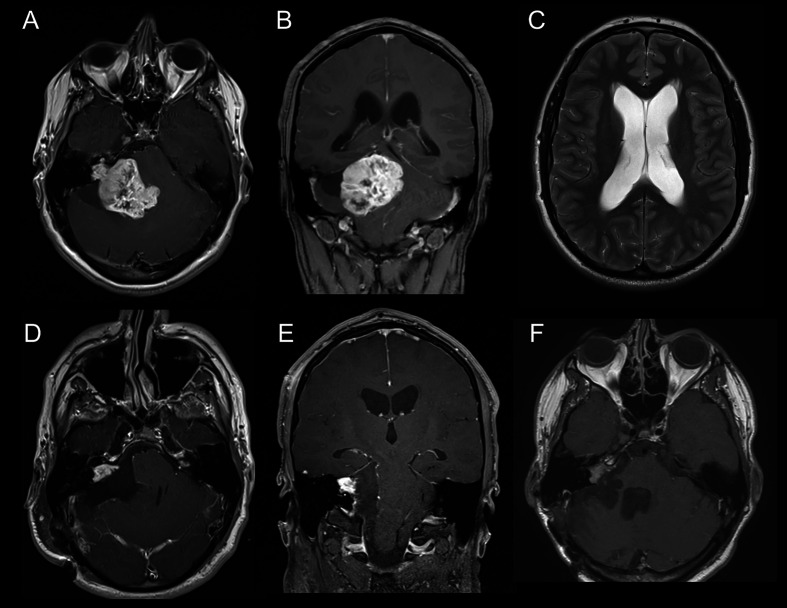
(A) Axial slice of MRI-T1Gd showing vestibular schwannoma KOOS IV on the right side. (B) Coronar slice of MRI-T1Gd. (C) Axial slice of MRI-T2 showing hydrocephalus with Evan´s ratio >0.3. (D) Axial slice of postoperative MRI-T1Gd showing residual tumor in the meatus acousticus internus. (E) Coronar slice of postoperative MRI-T1Gd. (F) Follow-up Image of MRI-T1Gd showing stable residual tumor after adjuvant radiotherapy.
